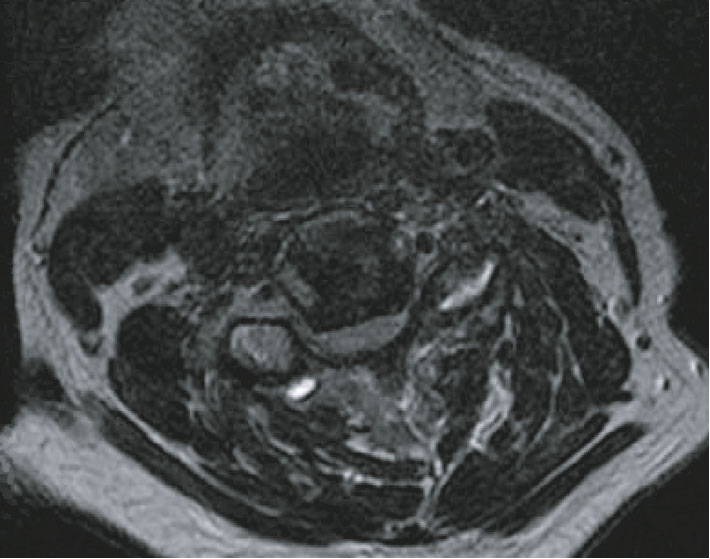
Axial T2-weighted MRI of the cervical spine, demonstrating severe canal stenosis with cord compression at the level of C4-C5.
